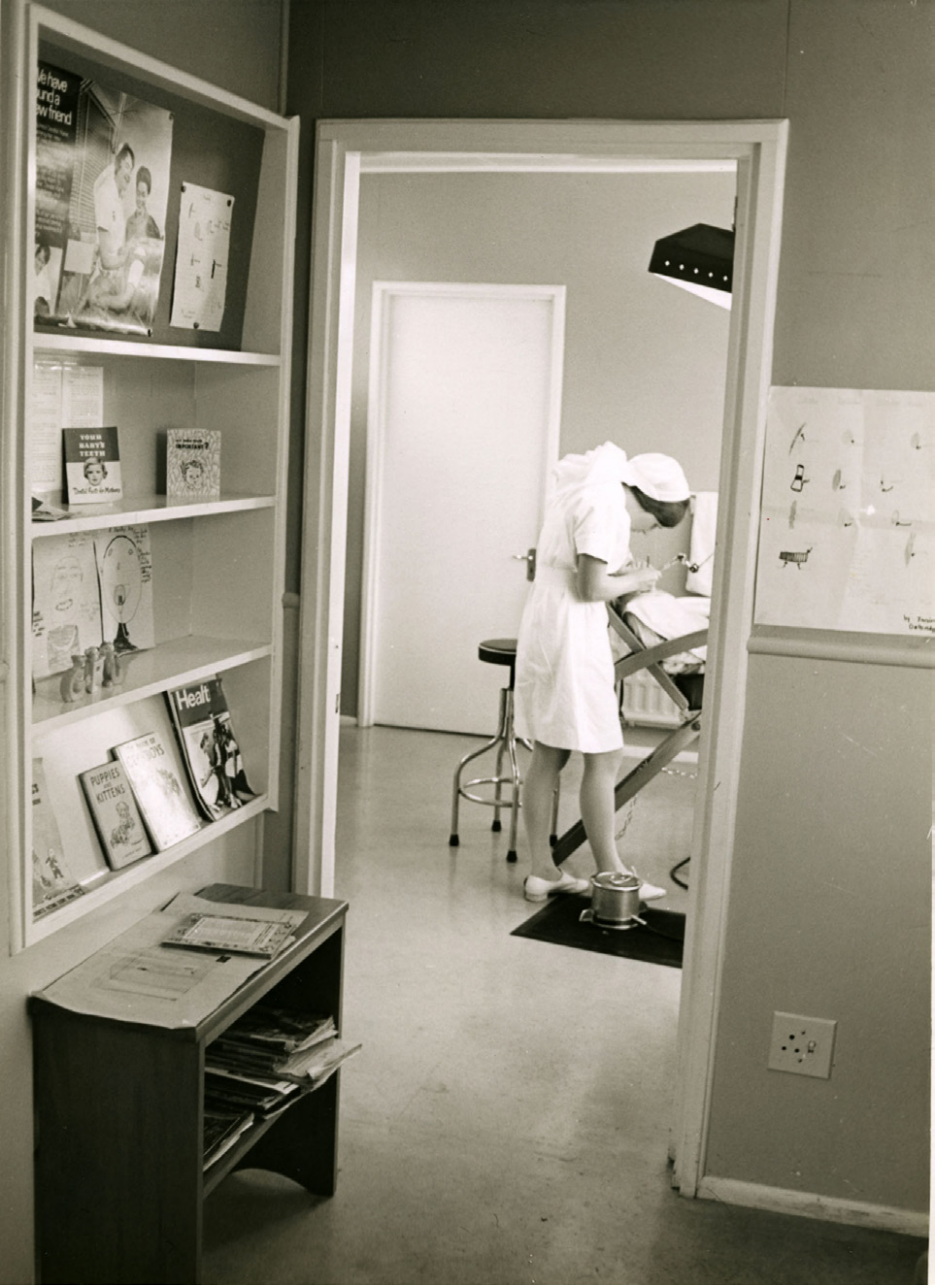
Dental clinic and dental nurse, 1970s [Archives New Zealand/Te Rua Mahara o te Kawanatanga, Wellington Office (Archives Reference: ABKI W4078 667/3)]. Cuts to health funding from the late 1970s meant that the School Dental Service had difficulties in keeping up-to-date with modern procedures, e.g., the introduction of block anesthesia and radiography, and less money was available for new dental materials and modern equipment. Poor working conditions and a lack of career progression would lead to dissatisfaction within the workforce. What had once been an innovative service was rapidly becoming outdated (81).
